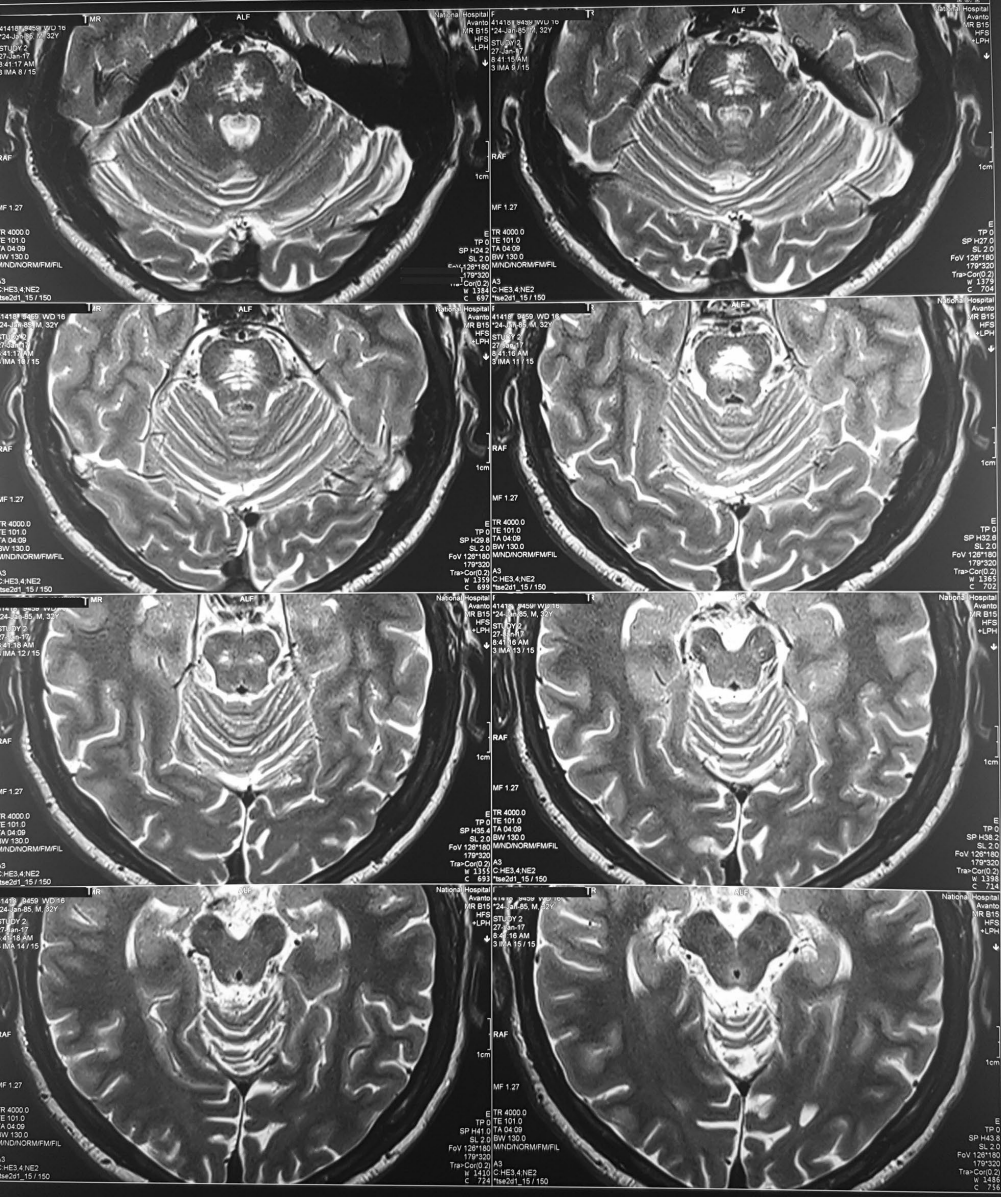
MRI of the brain stem, done 1 month after the first MRI showing signal intensities in FLAIR localized to the pons
